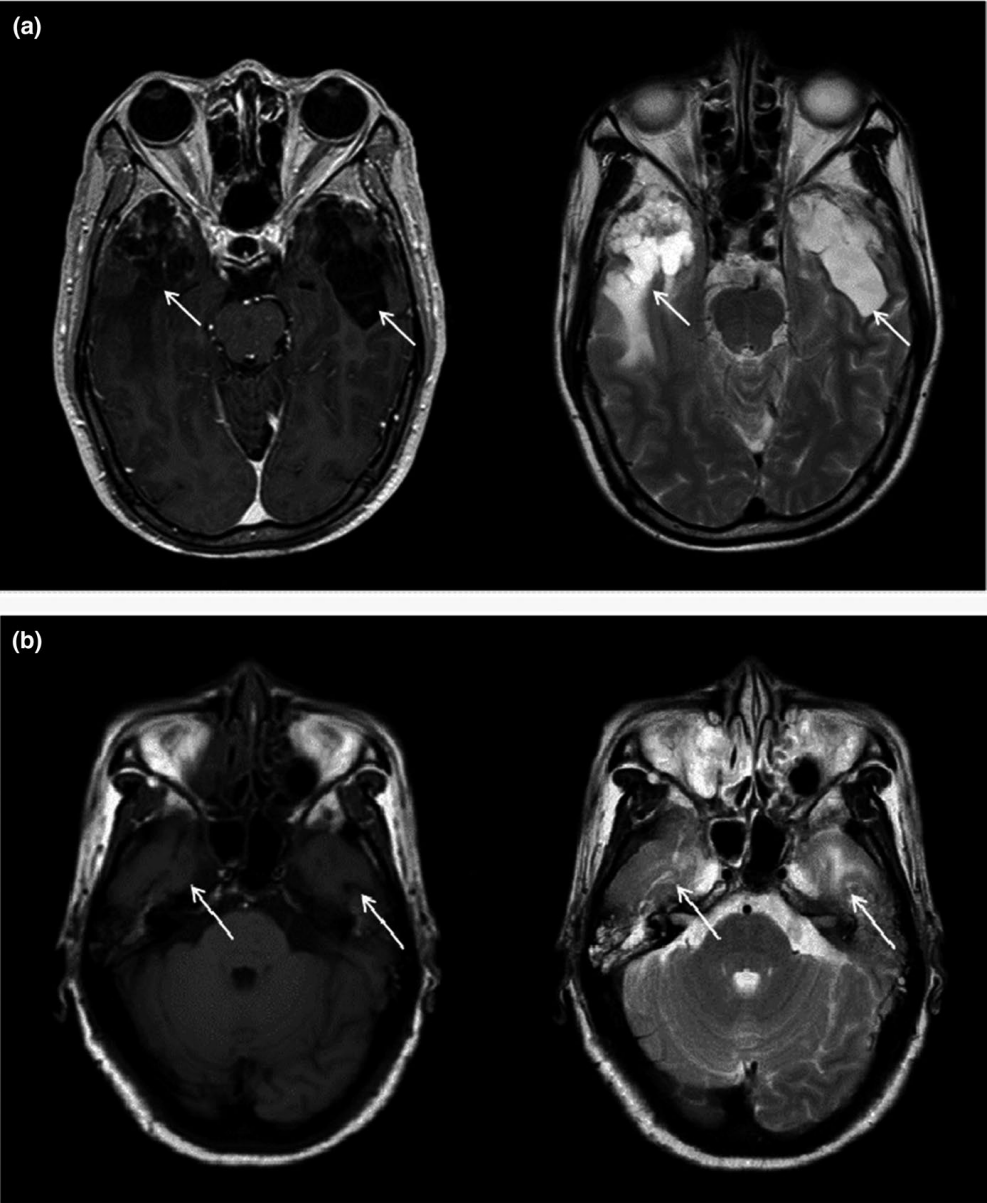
Representative MRI images of a patient with RBI. (a) T2‐weighted image showing finger‐like edema with bilateral lesions in temporal lobe and gadolinium‐enhanced T1‐weighted image show spot‐like and strip‐like enhancements with the right side. (b) T2‐weighted image showing small patchy abnormal signal shadows in bilateral temporal lobes and T1‐weighted image of the same patient
